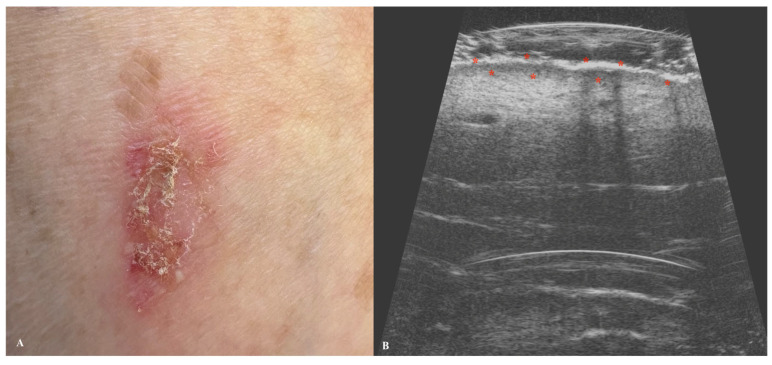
Macroscopic view of cutaneous lymphoma (A) with a corresponding ultrasonographic image (B). Stars indicate tumor margins.
